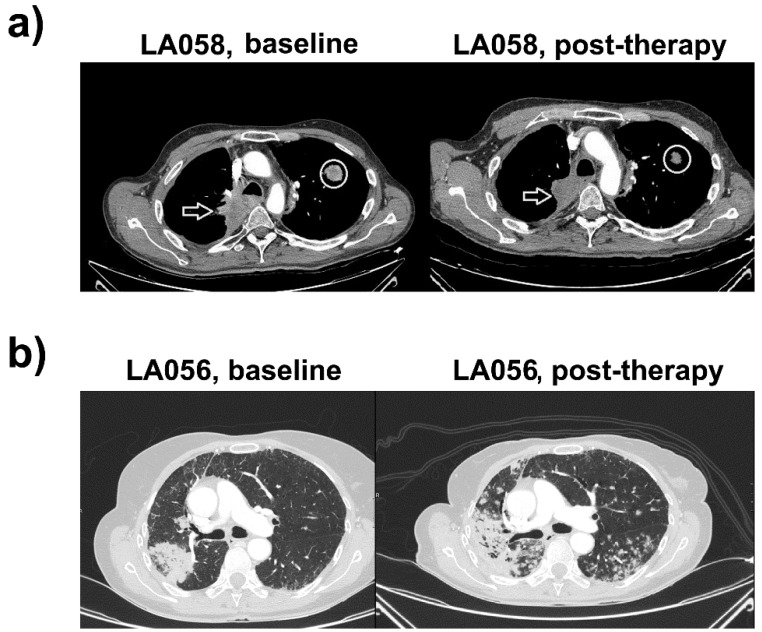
Clinical responses for tumor PD-L1-negative patients LA058 and LA056 treated with atezolizumab. (a) Left, CT images of LA058 before the start of immunotherapy. Right, CT images of LA058 after atezolizumab, demonstrating objective clinical responses, with evident tumor shrinkage. Arrows indicate a paravertebral upper right lobe mass with mediastinal invasion and posterolateral wall of the trachea. The circle indicates a contralateral metastatic node in the upper left lobe. Both lesions show a marked morphological decrease compatible with a partial response. (b) Left, CT evaluation of the LA056 patient. A tumoral relapse in the site of previous surgical intervention is evident. Right, CT following atezolizumab treatment. Tumor progression with bilateral pulmonary nodes and increase of the lower right lobe (LRL) mass, consistent with fast progressive disease.
